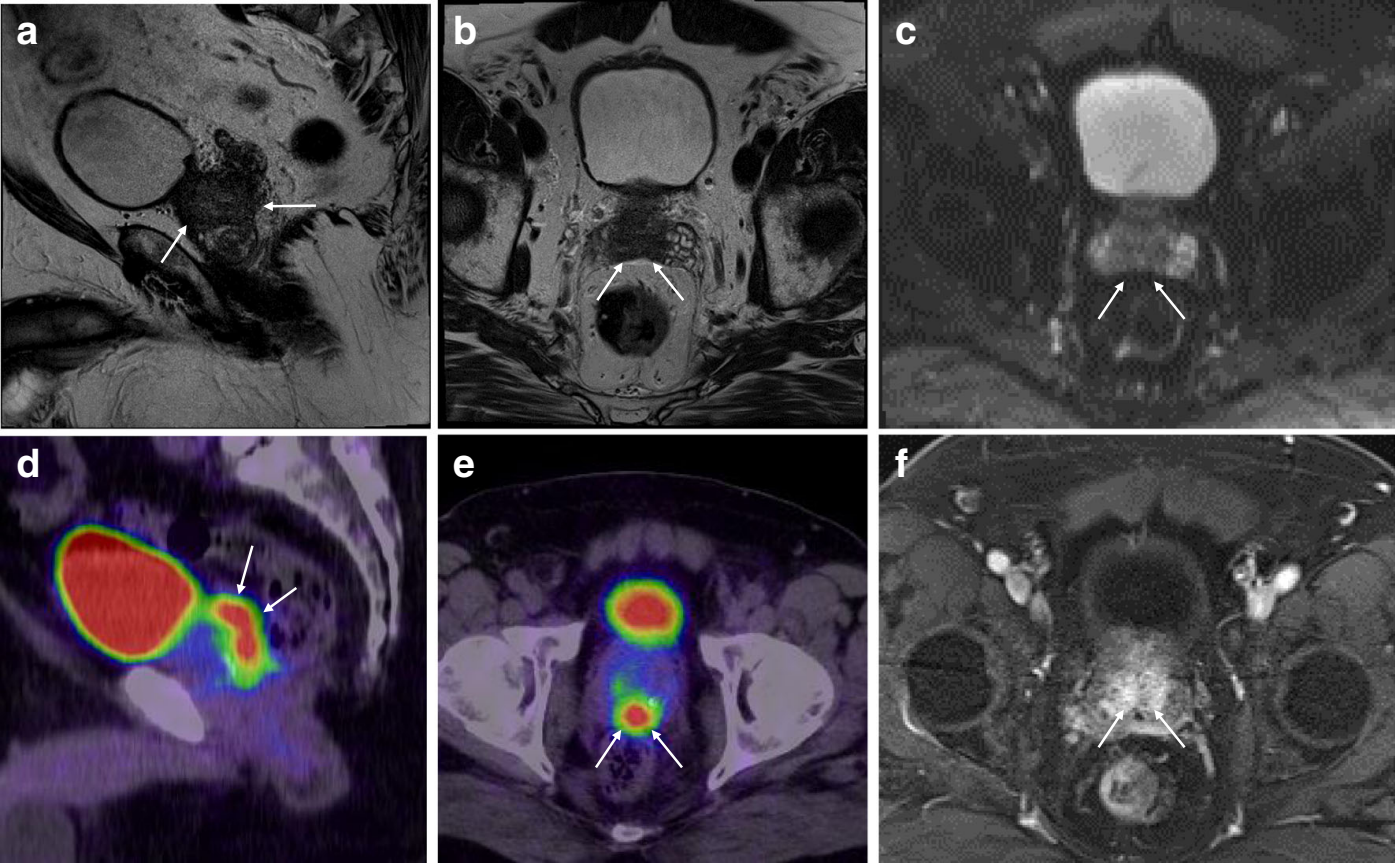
A 68-year-old patient presented a large extension of tumor mass in the base of the prostate gland with infiltration of the semilunar vesicles. In MP-MRI, this presents with a signal drop in T2w sequence (a, sagittal view; b, axial view), contrast enhancement in T1w sequence (f) and diffusion restriction, as demonstrated in the ADC map (c). PSMA-PET (*d*
